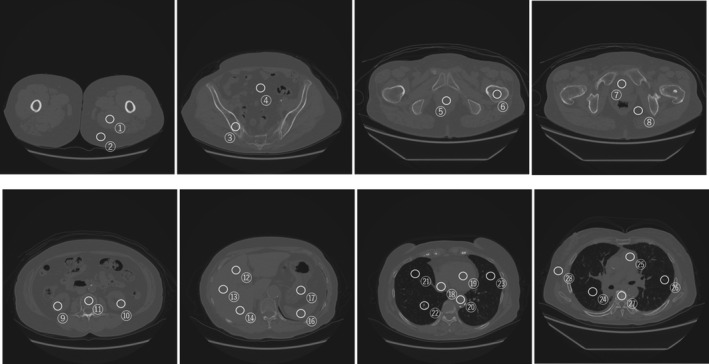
Measurement of the monoenergetic CT number from the feet‐to‐chest slice. Average and SD values of the monoenergetic CT number were measured by creating a circular ROI of 2 cm in diameter.
